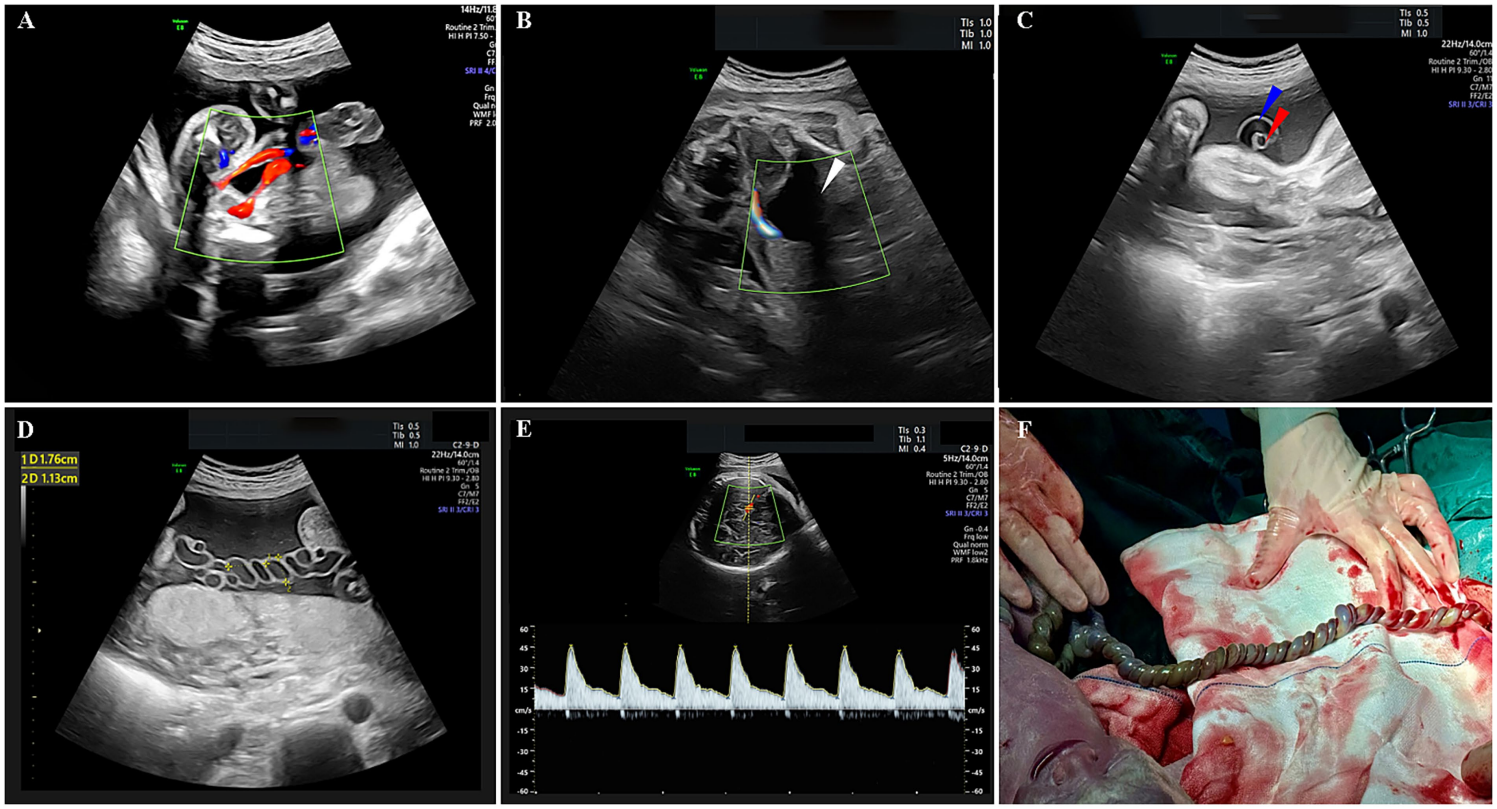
Prenatal ultrasound and post-delivery gross findings. (A) Prenatal ultrasound showing umbilical artery blood flow on both sides of the bladder at 23 3/7 weeks of gestation. (B) Prenatal ultrasound showing umbilical artery blood flow on only one side of the bladder at 32 1/7 weeks of gestation. (C) One umbilical artery with umbilical vein in cross-section. (D) Hypercoiling of the umbilical cord. (E) Blood flow of the middle cerebral artery. (F) Hypercoiled umbilical cord with focal discoloration. An umbilical cord measuring 90 cm in length with over 50 coils was observed. The white arrow indicates the bladder, the blue arrow indicates the umbilical vein, and the red arrow indicates the umbilical artery.
